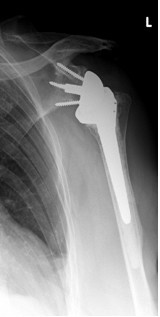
Antero-posterior radiograph of a 71 year-old female patient ´ s left shoulder with an implanted inverted total shoulder prosthesis Delta at 31 months of follow-up. Radiological analysis reveals "grade 4 = erosion over the inferior screw with extension under the base plate" of infraglenoidal scapular notching according to Nerot.
